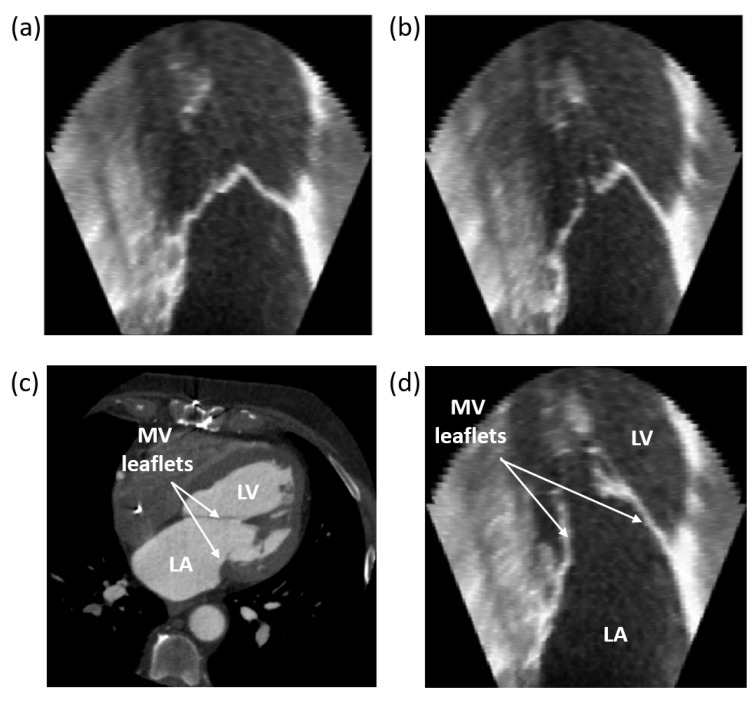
Results of the US frames selection related to one cardiac cycle. Frame selection related to the beginning (a) and to the end (b) of the cardiac cycle. The CT (c) and US (d) frame comparison operations approximately match in time, as confirmed by the MV leaflets opening in both volumes.
